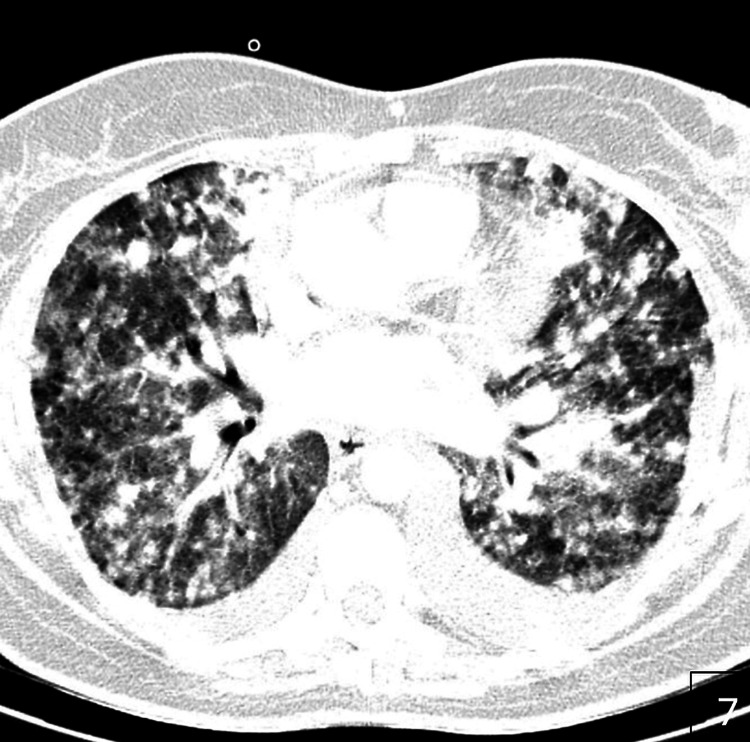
Axial CT scan demonstrating massive pulmonary metastases from renal cell carcinoma.
